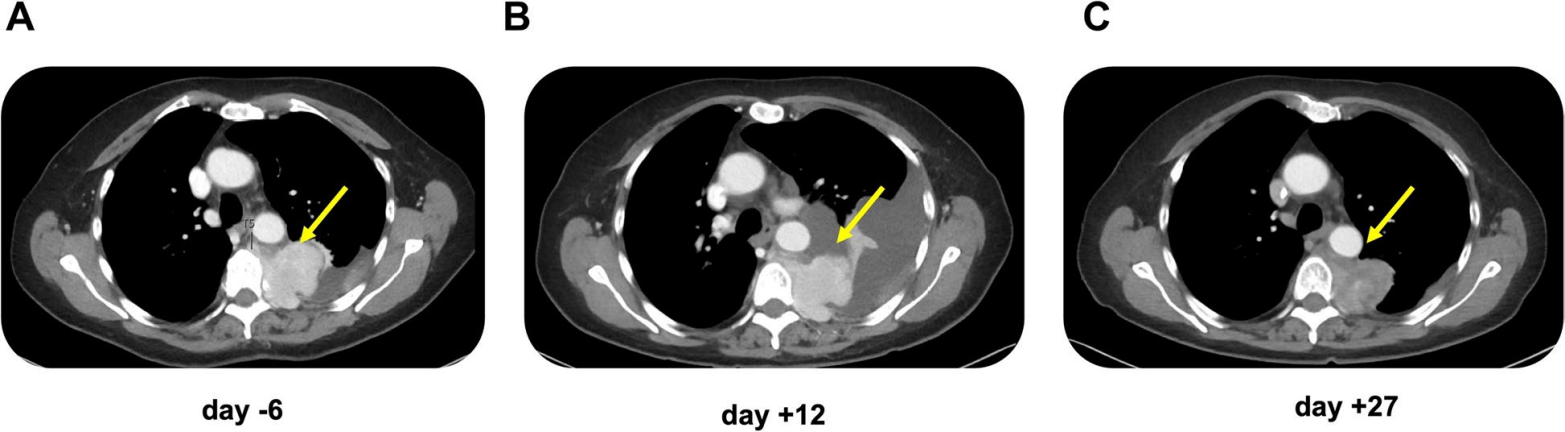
Radiographical response of extramedullary disease after ide-cel treatment in index patient with pleural extramedullary disease. CT scans (transversal) showing radiographical disease course at day -6 (A), + 12 (B) and + 27 (C) after ide-cel infusion. At day + 12 an initial increase in pleural infiltrative mass and pleural effusion is observed, followed by decrease of both at day + 27
